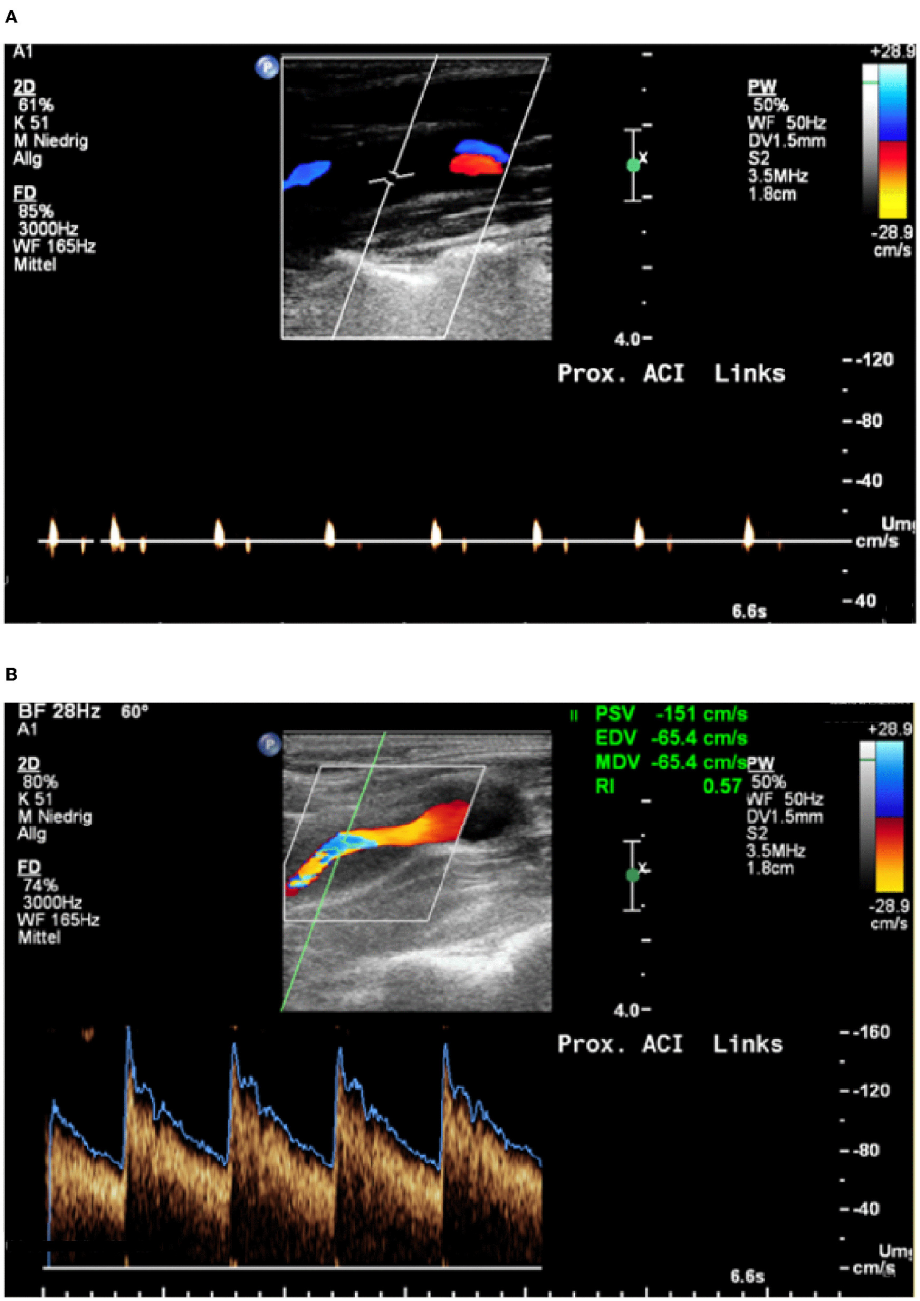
(A) 42-year-old patient with transient aphasia, paresthesia on the right, Horner syndrome on the left; Occlusion signal indicates occlusion of the internal carotid artery. (B) Neurovascular ultrasound done 3 days later shows recanalization with evidence of a wall hematoma.
